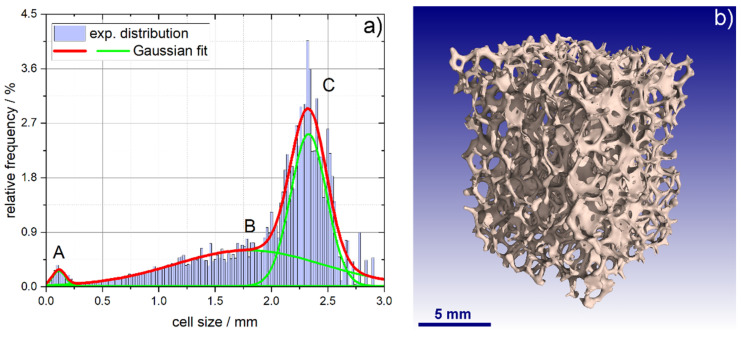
(a) structure separation distribution obtained from morphological analysis of µ-CT data on alumina foams; the maxima correspond to the hollow strut cavities (A), the cell windows (B; ~1.8 mm) and the actual foam cells (C; ~2.3 mm); (b) 3D rendering of the foam structure obtained from µ-CT data.
